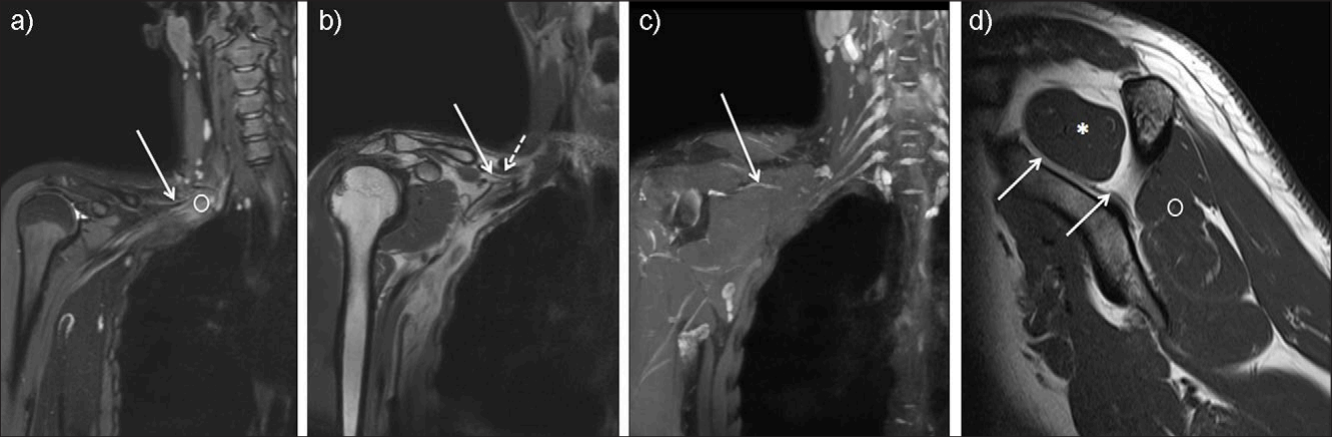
Coronal MR Neurography sections showing the right suprascapular nerve (solid arrow) arising from the upper trunk of the plexus brachial (circle) (2a = FIGURE 3 uploaded online manuscript) then, crossing the posterior cervical triangle in the supraclavicular fossa, deep to the omohyoid muscle (dashed arrow) (2b = FIGURE 4 uploaded online manuscript), before traveling through the suprascapular notch (2c = FIGURE 5 uploaded online manuscript). Sagittal MRI T1-weighted sequence shows the nerve inside the supraspinatus fossa where it provides motor innervation to the supraspinatus muscle (star) followed by infraspinatus muscle (circle) (2d = FIGURE 6 uploaded online manuscript).
